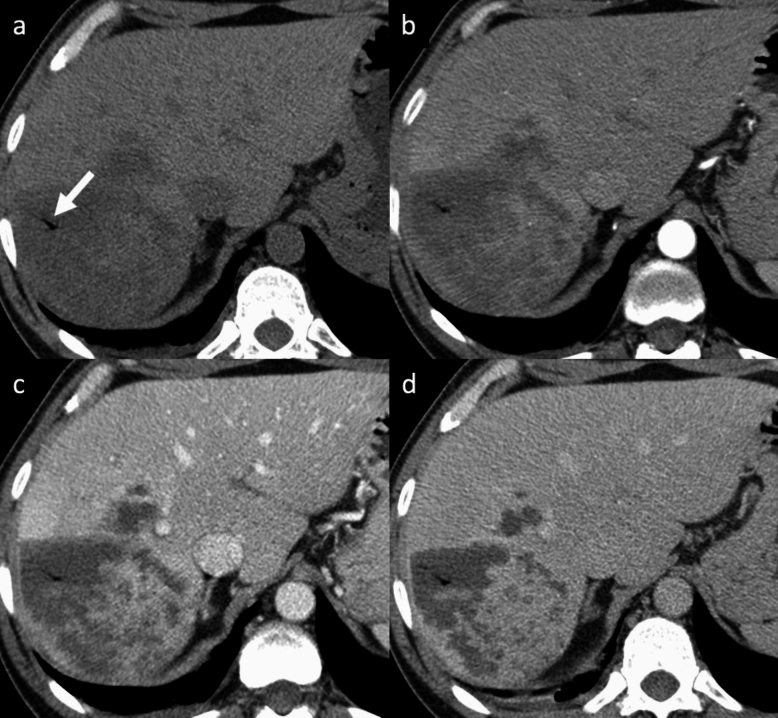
Liver abscess in a 48-year-old man with fever, jaundice and abdominal pain. a Axial unenhanced CT shows a hypoattenuating mass with irregular internal septa in the right hepatic lobe. Pneumobilia is visible inside the lesion (arrow, a). Contrast-enhanced CT shows a thick peripheral enhancement on arterial phase (b), that persists and progresses on portal venous (c) and delayed (d) phases
